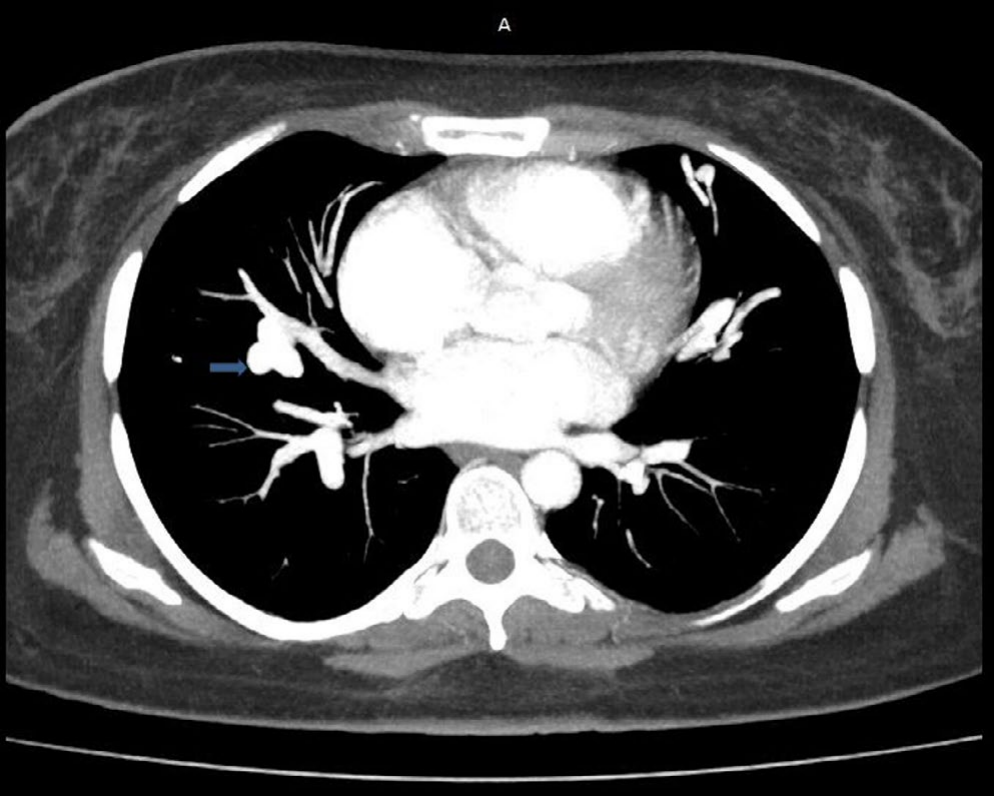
Mediastinal window chest CT scan with injection: pulmonary arteriovenous malformation of right pulmonary field
